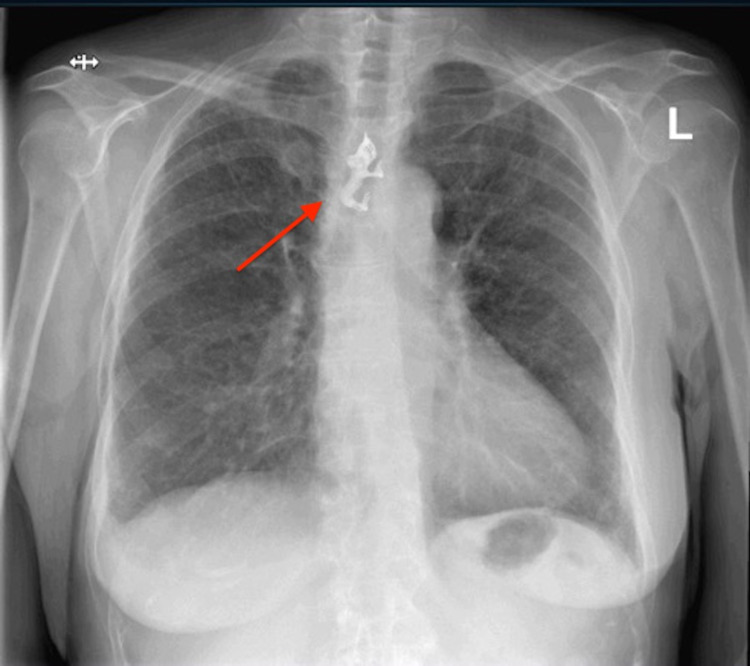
A chest radiograph demonstrating a radiopaque metallic dental bridge which is swallowed and trapped in the proximal third of the thoracic esophagus. Arrow pointed at the foreign body.
